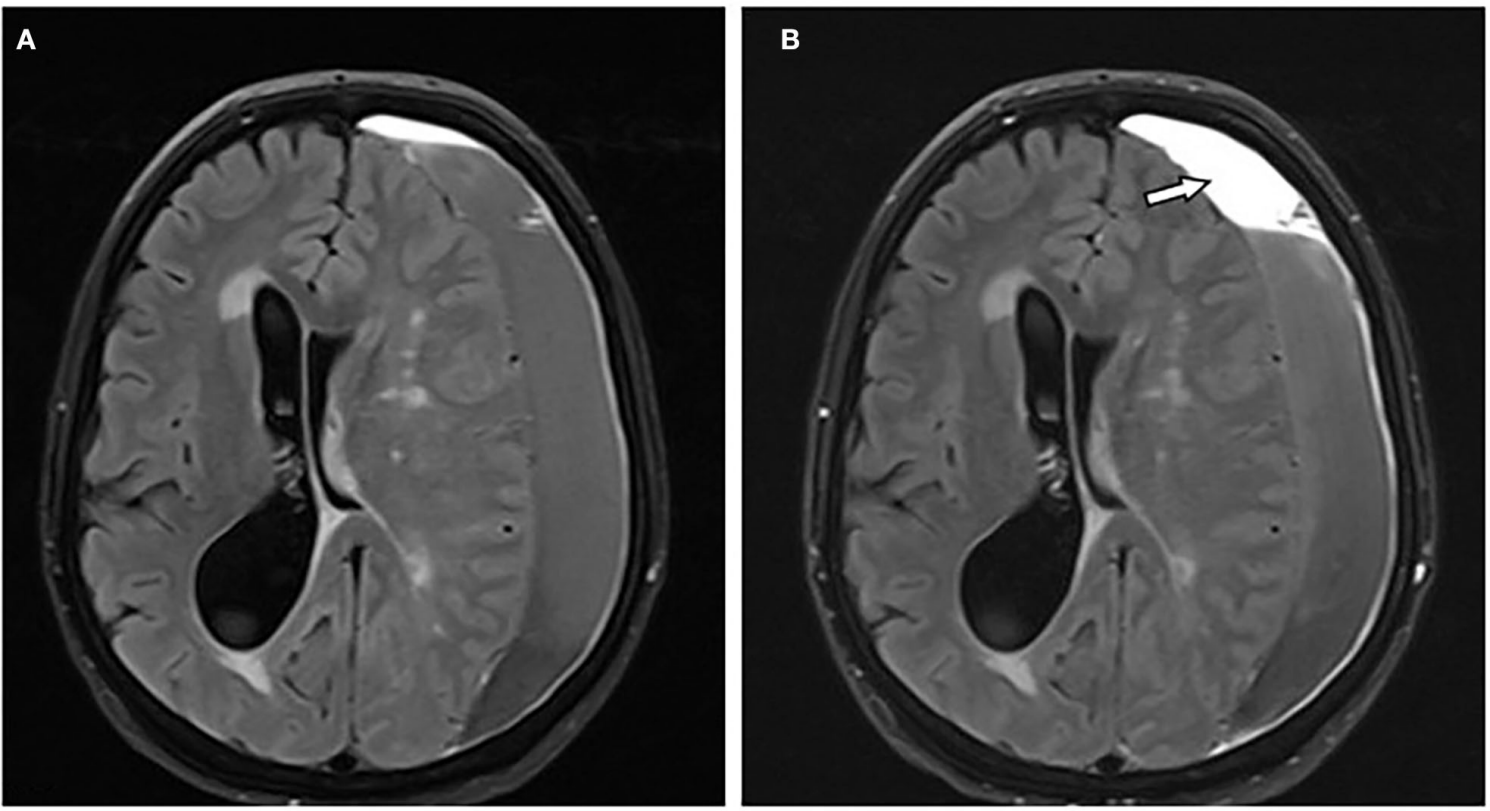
A 66-year-old female patient with chronic subdural hematoma (cSDH) over the left convexity. Axial T2-FLAIR images before (A) and after (B) contrast administration showing mass-like enhancement of the hematoma [(B): arrow].
